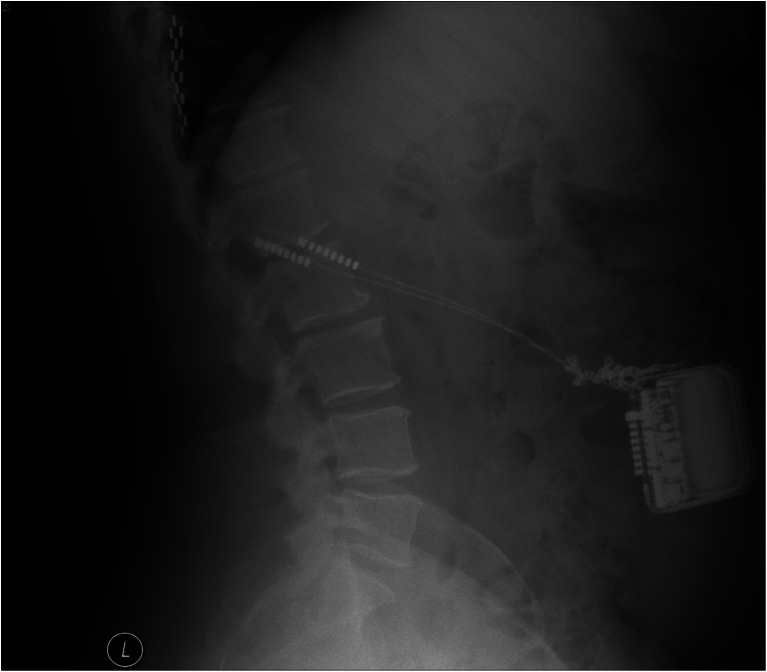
Lateral plain abdominal X-ray showing twisting of the connecting wires adjacent to the IPG
